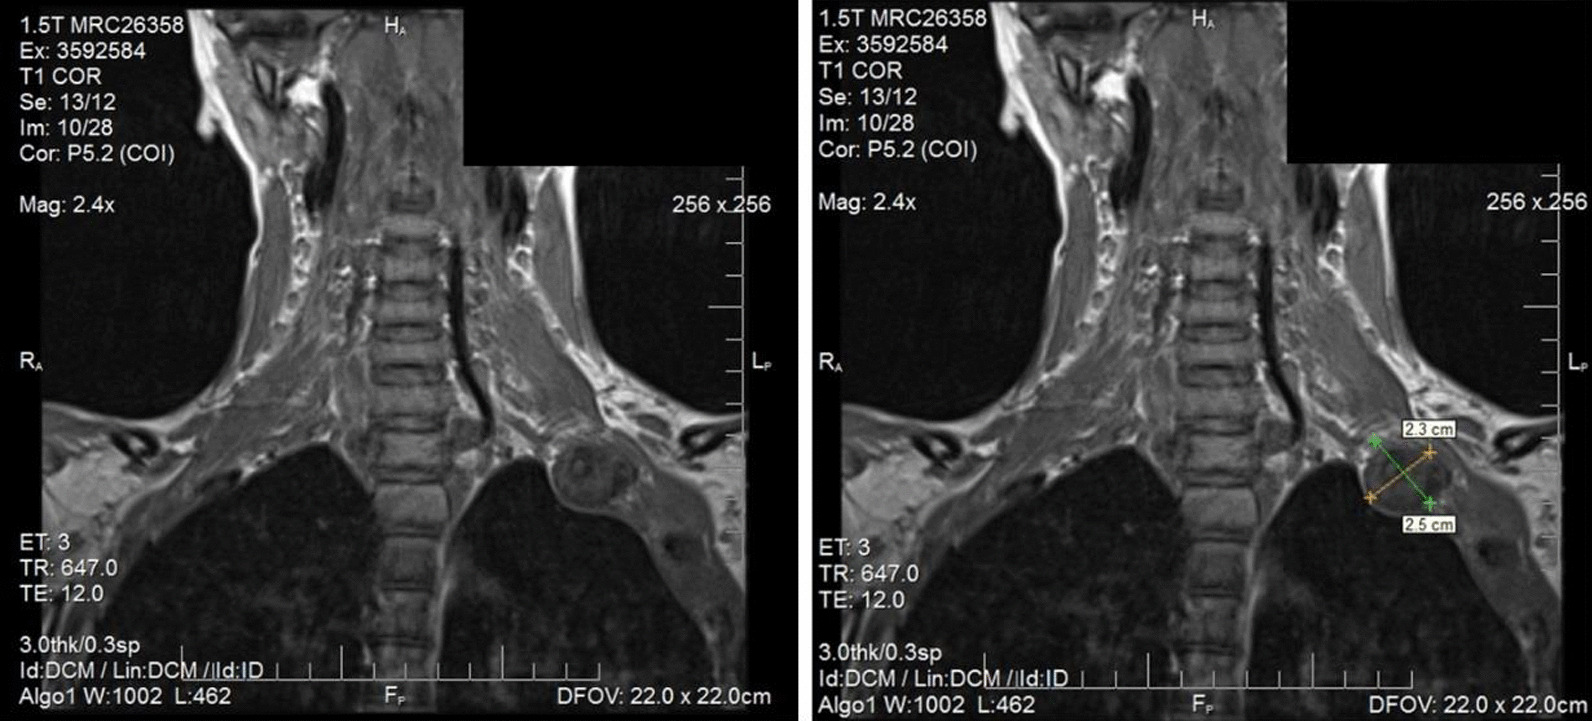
Coronal view of cervical magnetic resonance imaging (MRI) showing pseudoarthrosis of left first rib
